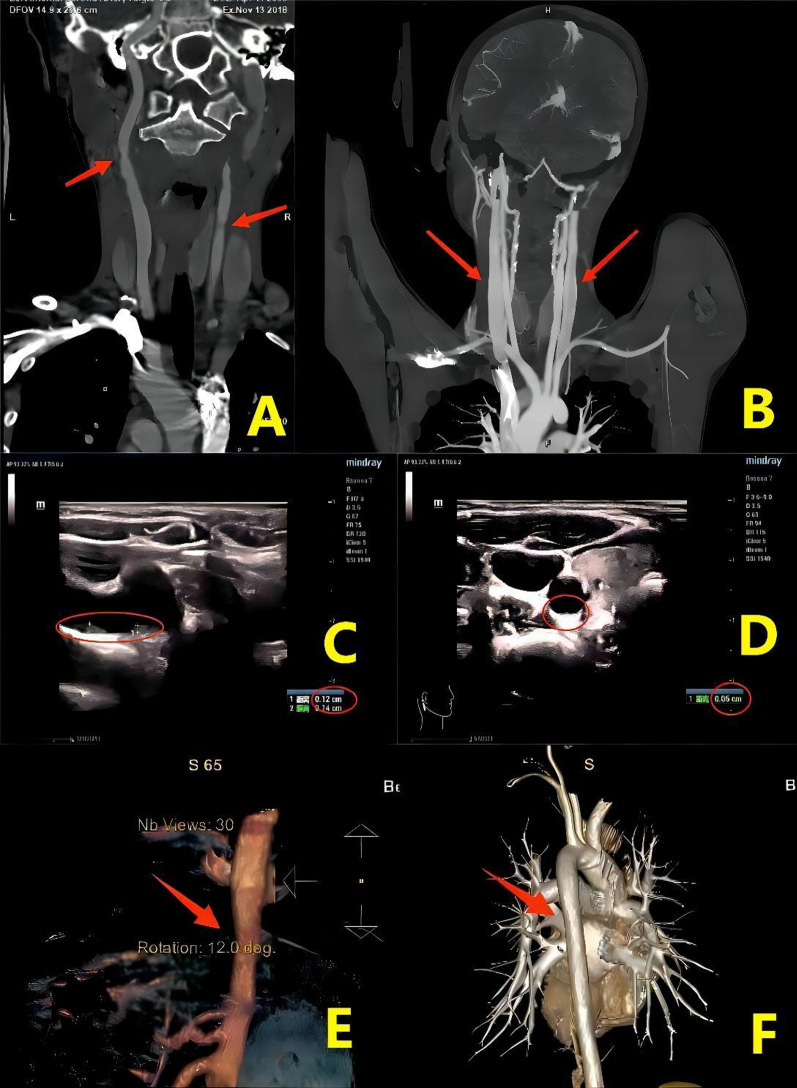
Vascular changes before and after liver transplantation in children. A Case 2 carotid artery stenosis (red arrow). B The recovered carotid artery in case 2 (red arrow). C In case 4, the preoperative carotid intima-media thickness was 0.12 cm (red circle). D Carotid artery intima-media thickness of case 4 was about 0.05 cm 3 years after operation (red circle). E In case 3, preoperative computed tomography (CT) showed descending aortic plaque (red arrow). F In case 3, postoperative CT showed that the descending aorta plaque disappeared (red arrow)
